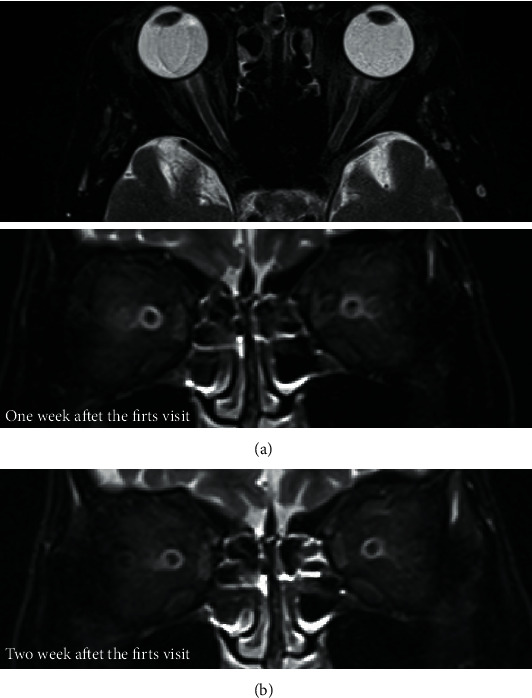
Magnetic resonance imaging (MRI). (a) One week after the first visit, T2-weighted MRI demonstrates well-marked enhancement of the left optic nerve compared to the right optic nerve. (b) Two months after the first visit, MRI showed improvement in the enhancement of the left optic nerve.
